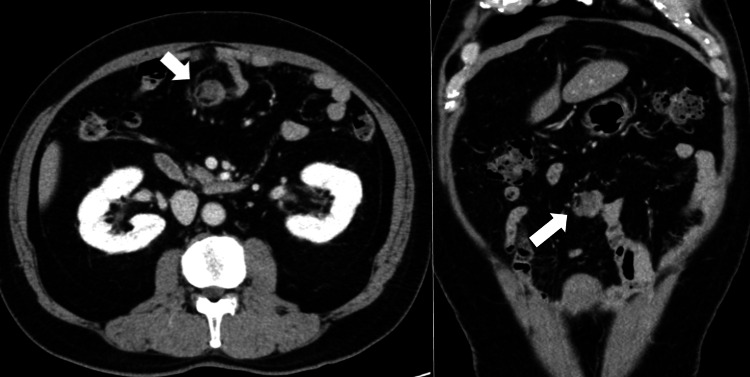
Contrast-enhanced CT scan of lesion 2, measuring 2 cm in diameter (white arrows). CT, computed tomography
